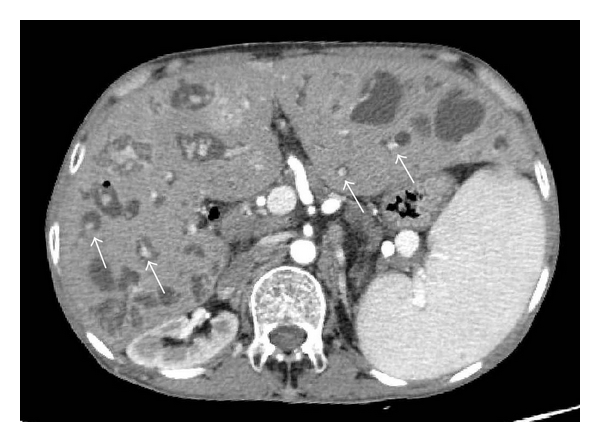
Dynamic CT reveals multiple cystic dilatations of the intrahepatic bile ducts in a patient with Caroli's disease with CHF. The arrows indicate the central dot sign.
